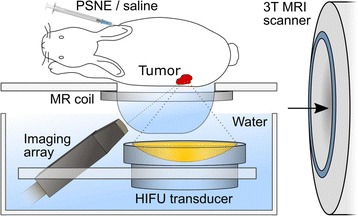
Setup for multi-modality imaging of focused ultrasound ablation
